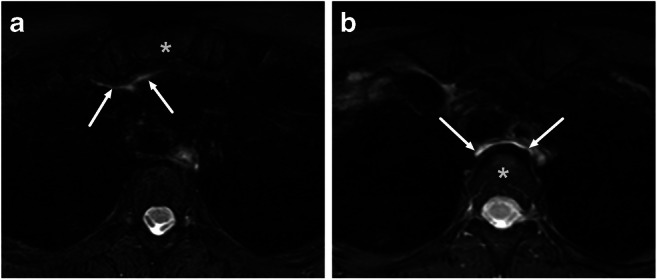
Examples of anterior (a) and posterior (b) mediastinal edema on axial fat-suppressed T2-weighted Dixon images. a A 46-year-old female with tonsillitis had edema in the anterior mediastinum at the level of the manubrium sterni (asterisk). b A 48-year-old female with peritonsillar and parapharyngeal abscesses had edema in the posterior mediastinum at the level of the Th3 vertebra (asterisk)
